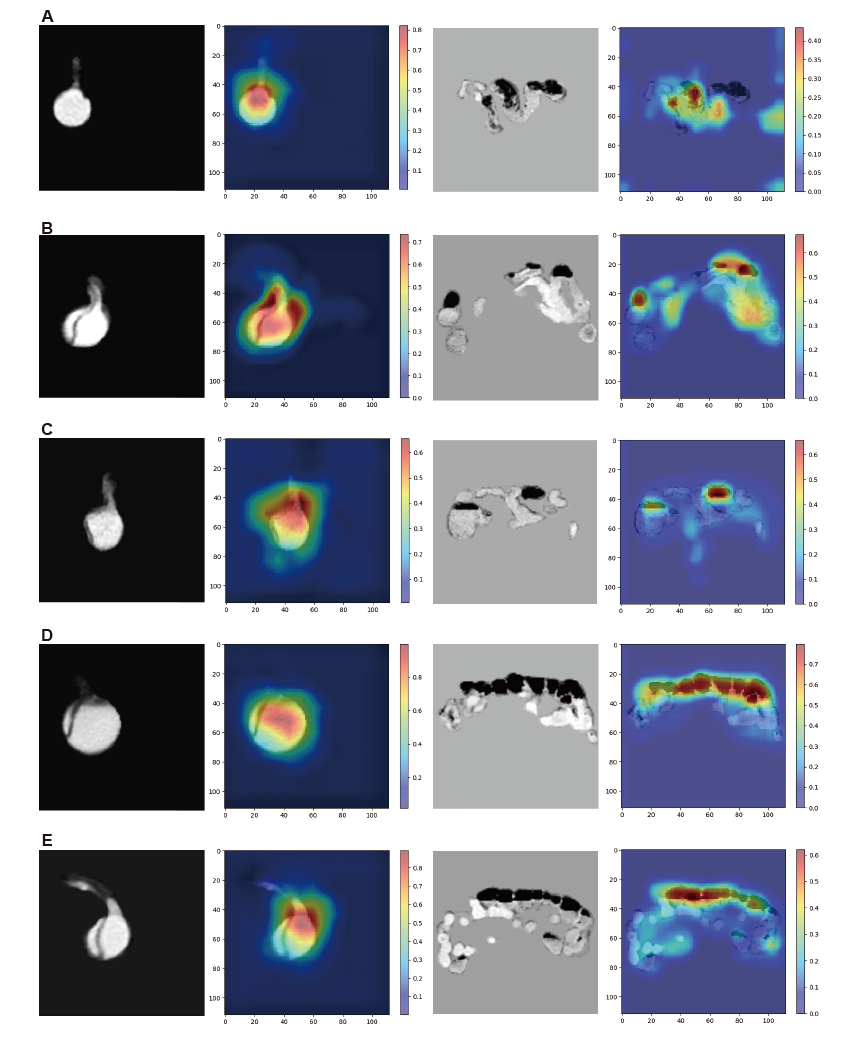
Attention maps of the multiorgan feature-based acute aortic dissection complicating mesenteric malperfusion model using gradient-weighted class activation mapping (Grad-CAM). Panels A-E display computed tomography angiography (CTA) images and attention maps generated by Grad-CAM for 5 randomly selected patients with mesenteric malperfusion. Each panel shows the abdominal aorta CTA cross-section on the left and the bowel CTA cross-section on the right. The red regions indicate high-weight areas critical to model decision-making, whereas the blue regions indicate areas with low weights.
